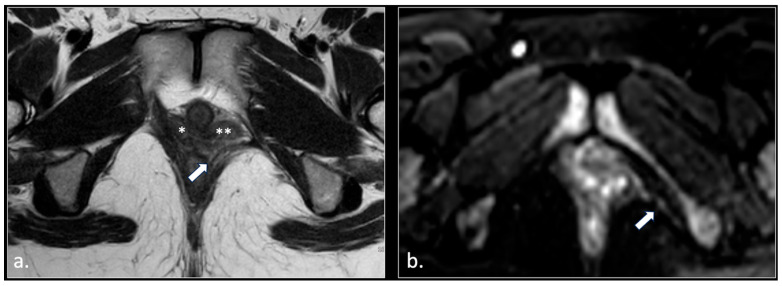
A 43-year-old female with chronic post-traumatic pudendalgia. (a) Axial T2-weighted scan shows a normal right side pubo-coccygeus muscle (*). On the left side, an avulsion of the pubo-coccygeus muscle can be seen. The residual left pubo-coccygeus muscle is thinned (arrow). The vagina is shifted on the left side (). (b) Axial b600 DWI scan demonstrates diffuse hyperintensity of the left pudendal nerve (arrow).
